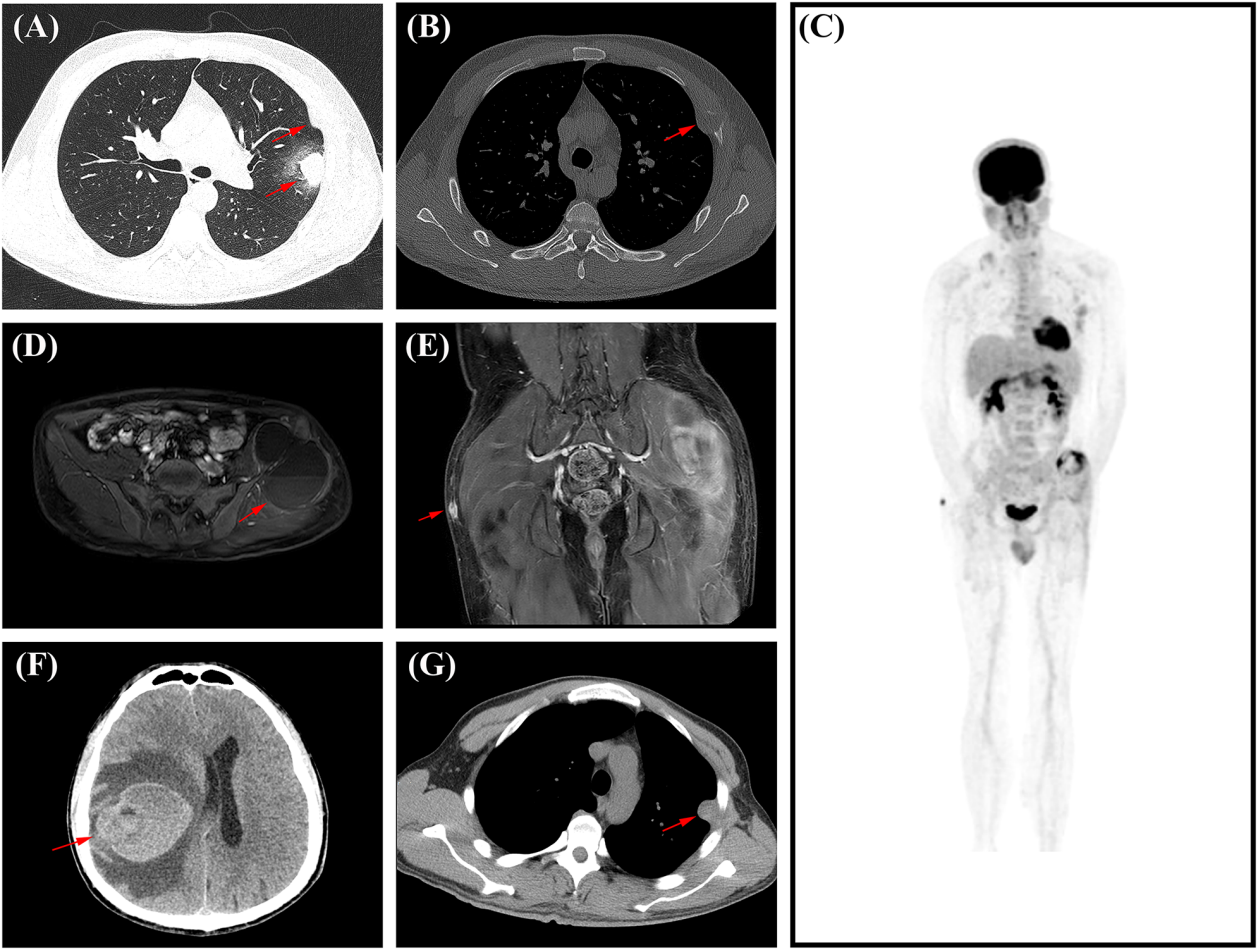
The imaging data of this patient’s disease progression. (A) In August 2022, chest CT showed a 2.8cm×2.1cm mass in the upper lobe of the left lung. (B) In August 2022, on chest CT, the red arrow refers to the left fourth rib lesion. (C) In May 2023, PET/CT showed multiple metastases throughout the body. (D) In May 2023, Axial T1 MRI contrast of the pelvis shows metastases in and around the left iliac bone, measuring 9.6 cm×6.4 cm, with a visible fluid-fluid level within it (arrow). (E) In May 2023, coronal T1 MRI enhancement showed a subcutaneous nodule on the right buttock, approximately 1.1 cm×1.0cm in size (arrow). (F) In October 2023, brain CT showed large patches of hyperdense opacities in the right basal ganglia and frontoparial lobe, with the maximum level ranging from about 6.2cm×5.0cm (arrow). (G) In October 2023, chest CT showed a soft tissue nodular opacity at the base of the left pleura, about 1.6 cm×2.6cm in size (arrow).
